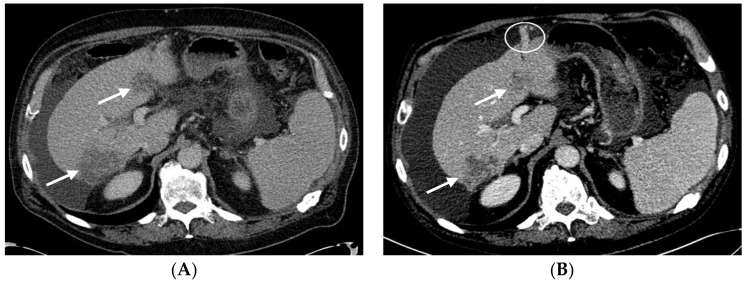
The tumors remained stable following cycle 9 and cycle 15 with CAPOX-B. (A) Following cycle 9 with CAPOX-B, the axial contrast-enhanced CT scans demonstrate stable heterogeneous hepatic tumors (arrows) compatible with a diagnosis of hepatocellular carcinoma. The right hepatic lobe tumor is subcapsular in location. There are small peri-hepatic and peri-gastric ascites. (B) Following cycle 15 with CAPOX-B, the axial contrast-enhanced CT scans demonstrate persistent heterogeneously enhanced hepatic tumors of a stable size (arrows). Progressive peri-hepatic and peri-gastric ascites with recanalized para-umbilical vein (circle) and splenomegaly can be seen.
